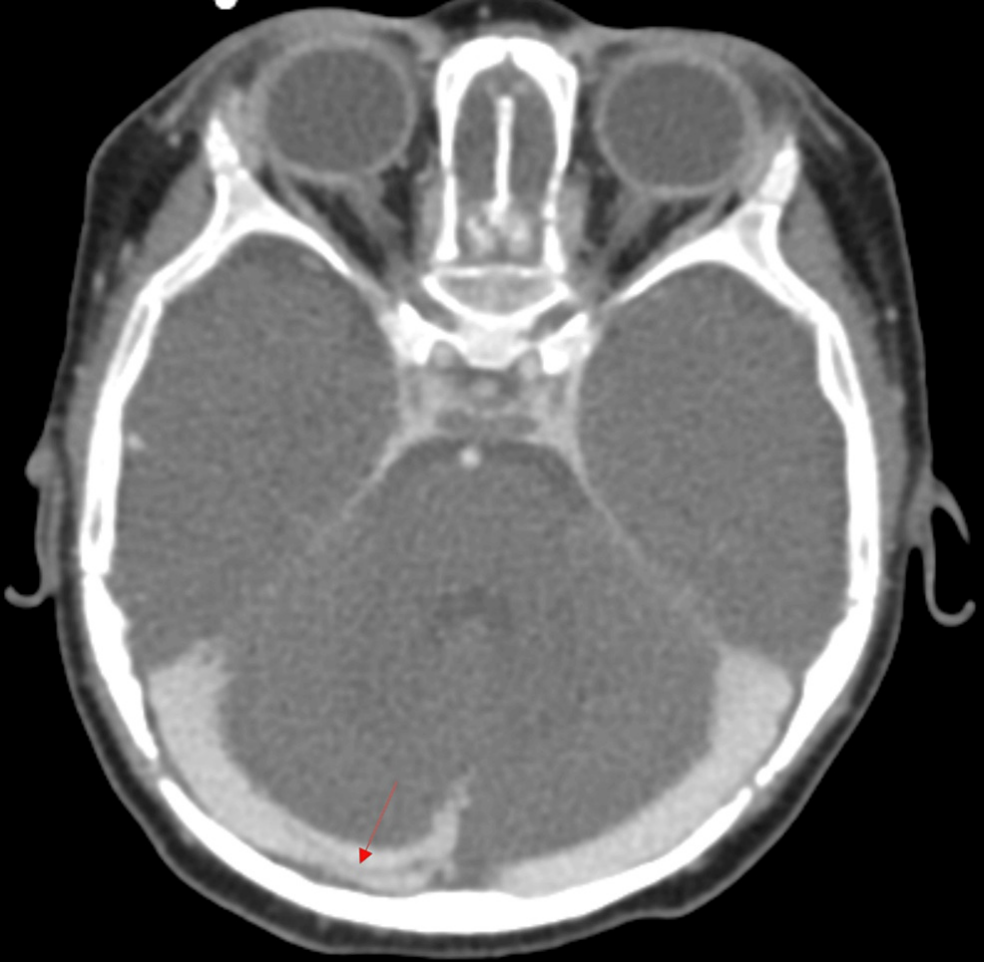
CT venogram of the cranial vault with contrast depicting a resolved thrombus (red arrow) in right transverse sinus.
